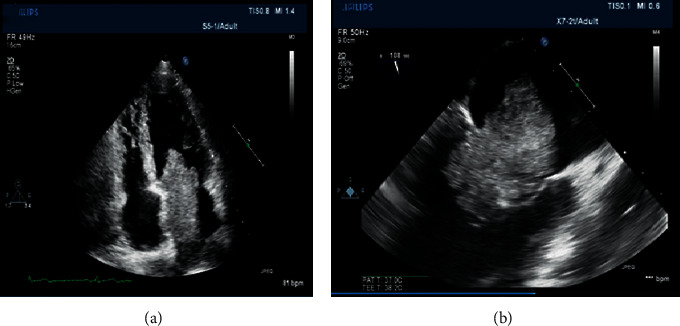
Transthoracic echocardiogram showing a large mass attached to the interatrial septum (a). The mass has a broad stalk and protrudes into the left ventricle during diastole and almost completely occludes the mitral valve (b).
